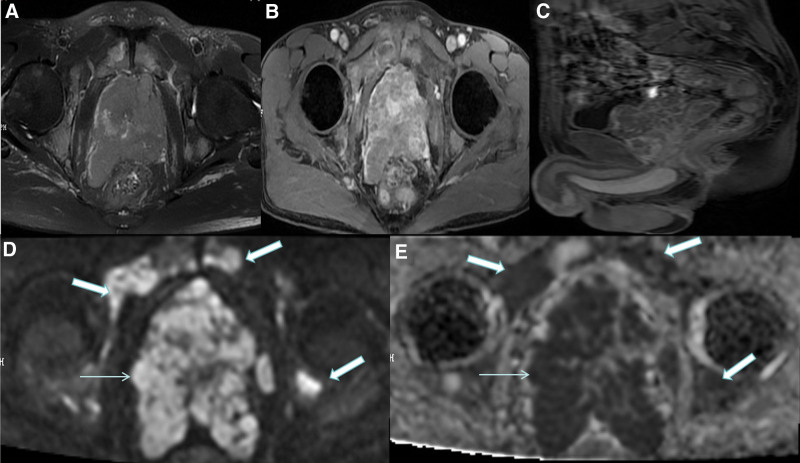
The prostate was significantly enlarged, irregular in shape, capsule destruction and adjacent structure invasion, T2WI signal of prostate was reduced, MRI showed the enhancement feature of fast forward and fast out, and multiple pelvic metastases (A–C). Prostate masses (blue arrow) and bone metastases (boxed blue arrow) were significantly limited in diffusion (D and E). MRI = magnetic resonance imaging.
